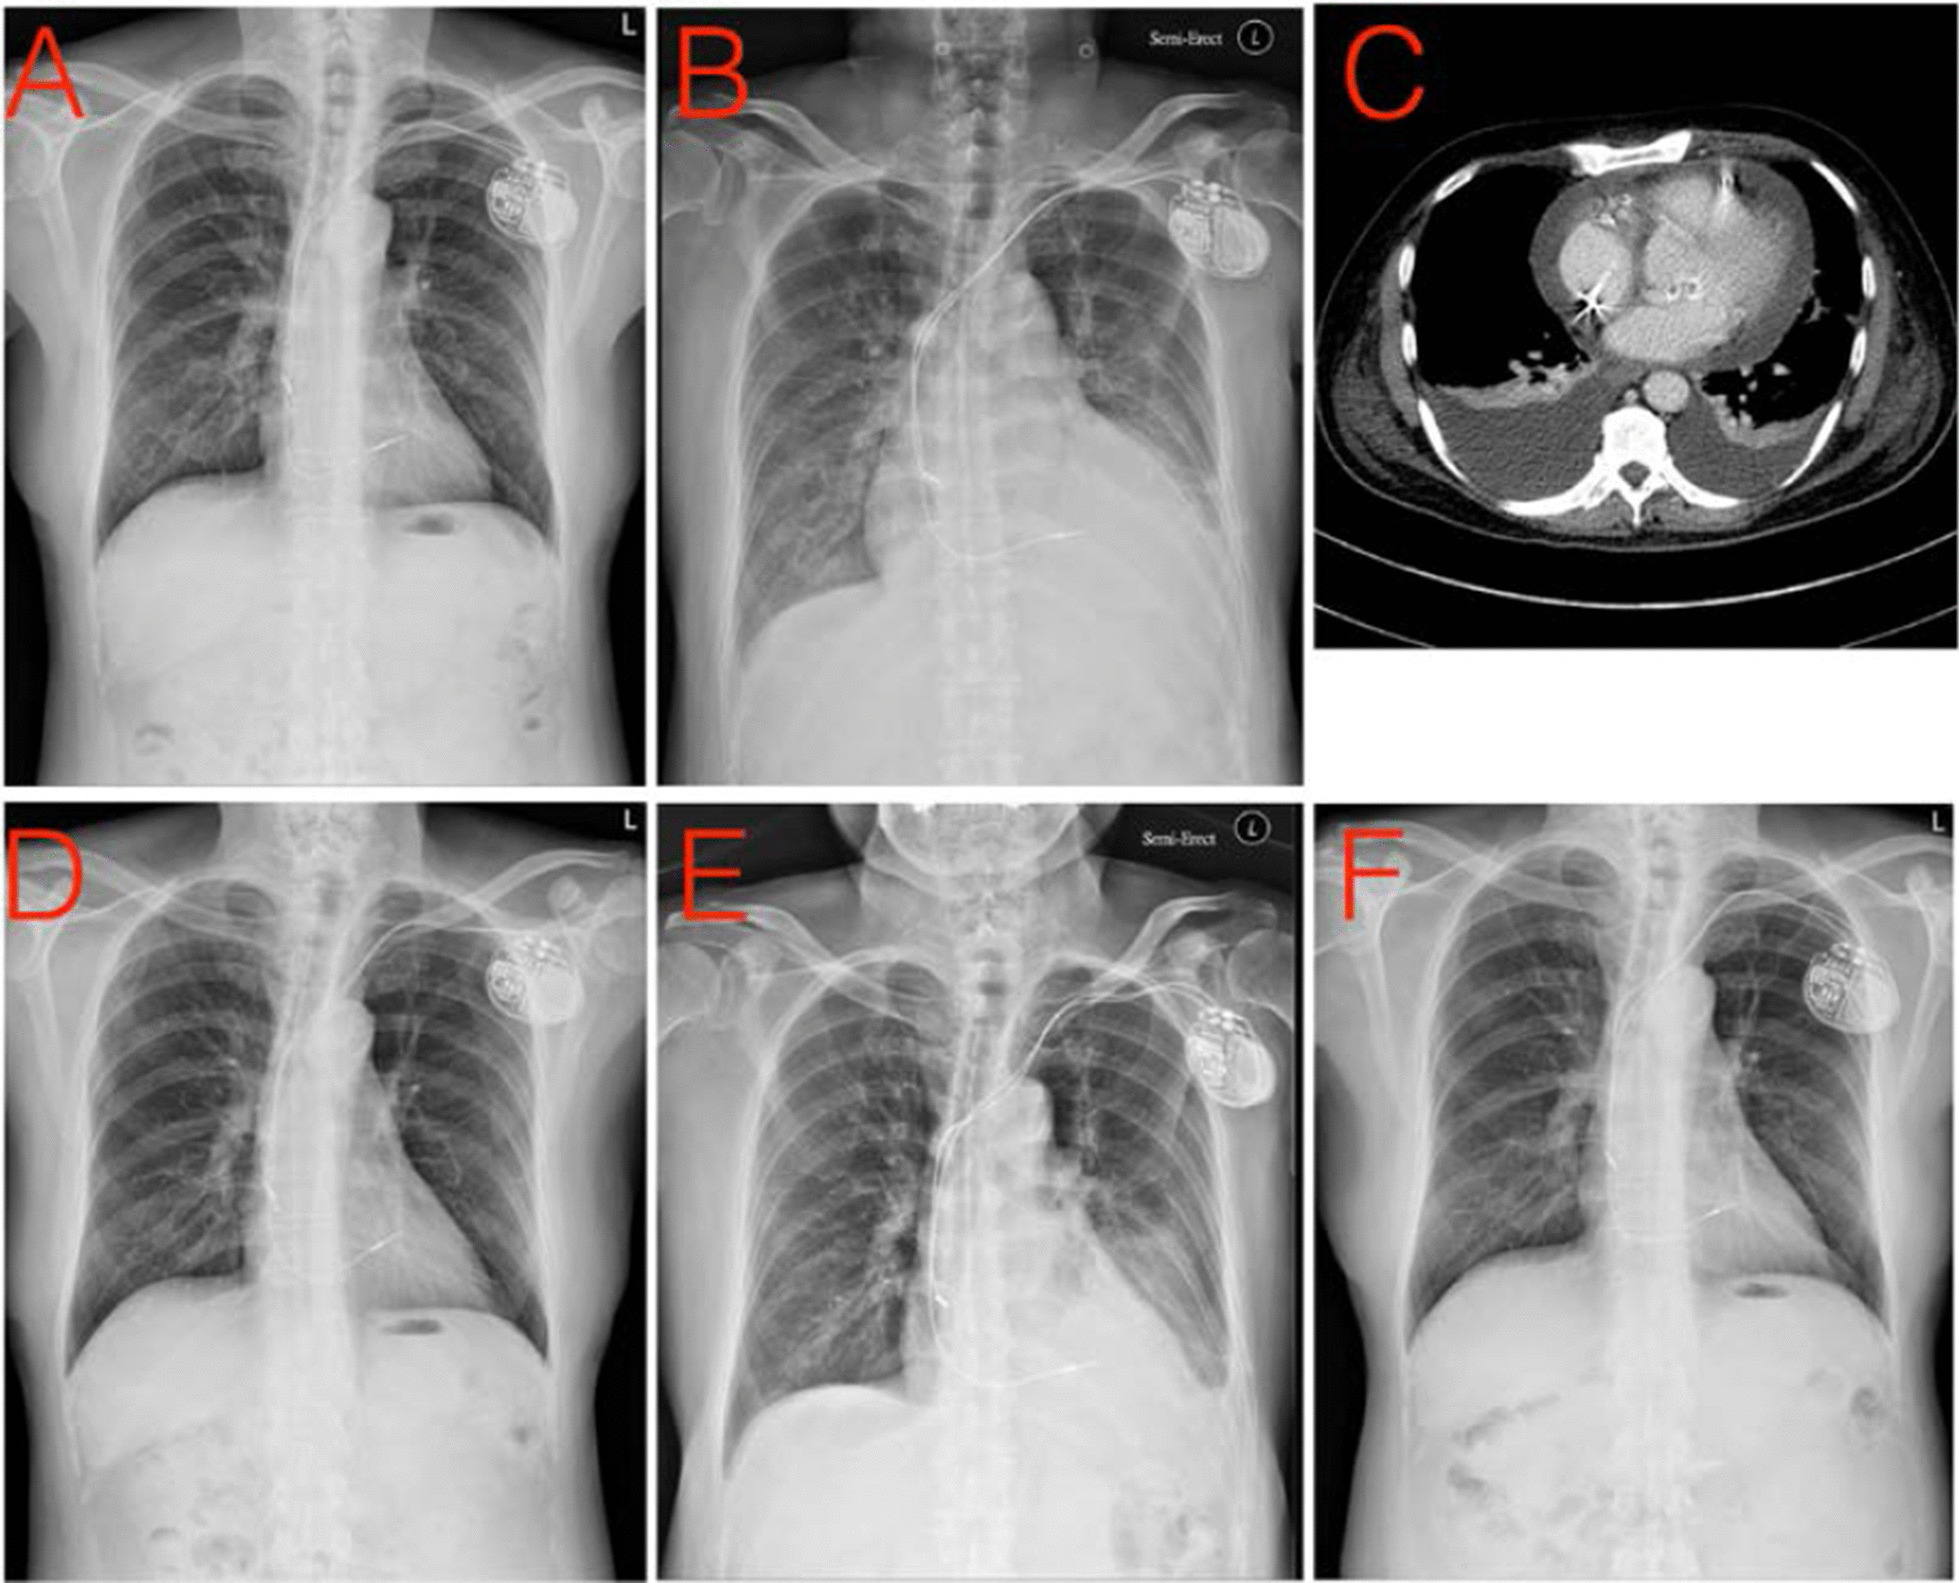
Case 1 series images. a One day after implantation of pacemaker; b significant increase in cardiothoracic ratio at fourteen days after implantation; c CT scan showed no lead perforation, no pericardial effusion or pleural effusion; d After steroid treatment; e Recurrent PCIS, two weeks after completion of the steroid course; f Fourteen days after treatment with steroids and colchicine
